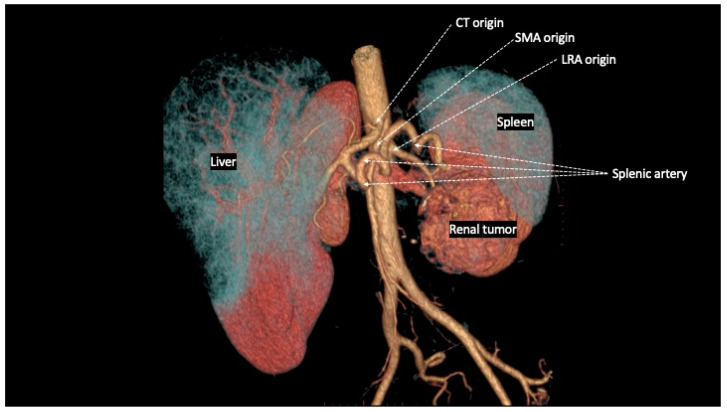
Three-dimensional reconstruction of vascular anatomy spatial relationships on computed tomography with intravenous contrast: the patient had combined SMA and splenic artery injuries during laparoscopic left radical nephrectomy for a large renal cell carcinoma with periaortic tissue involvement.
